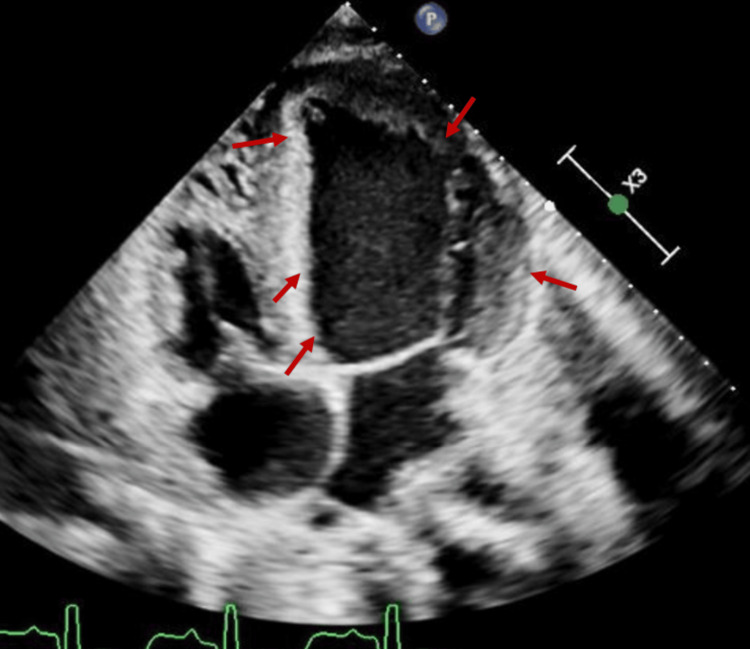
Apical view of a transthoracic echocardiogram showing a dilated left ventricle (red arrows)
